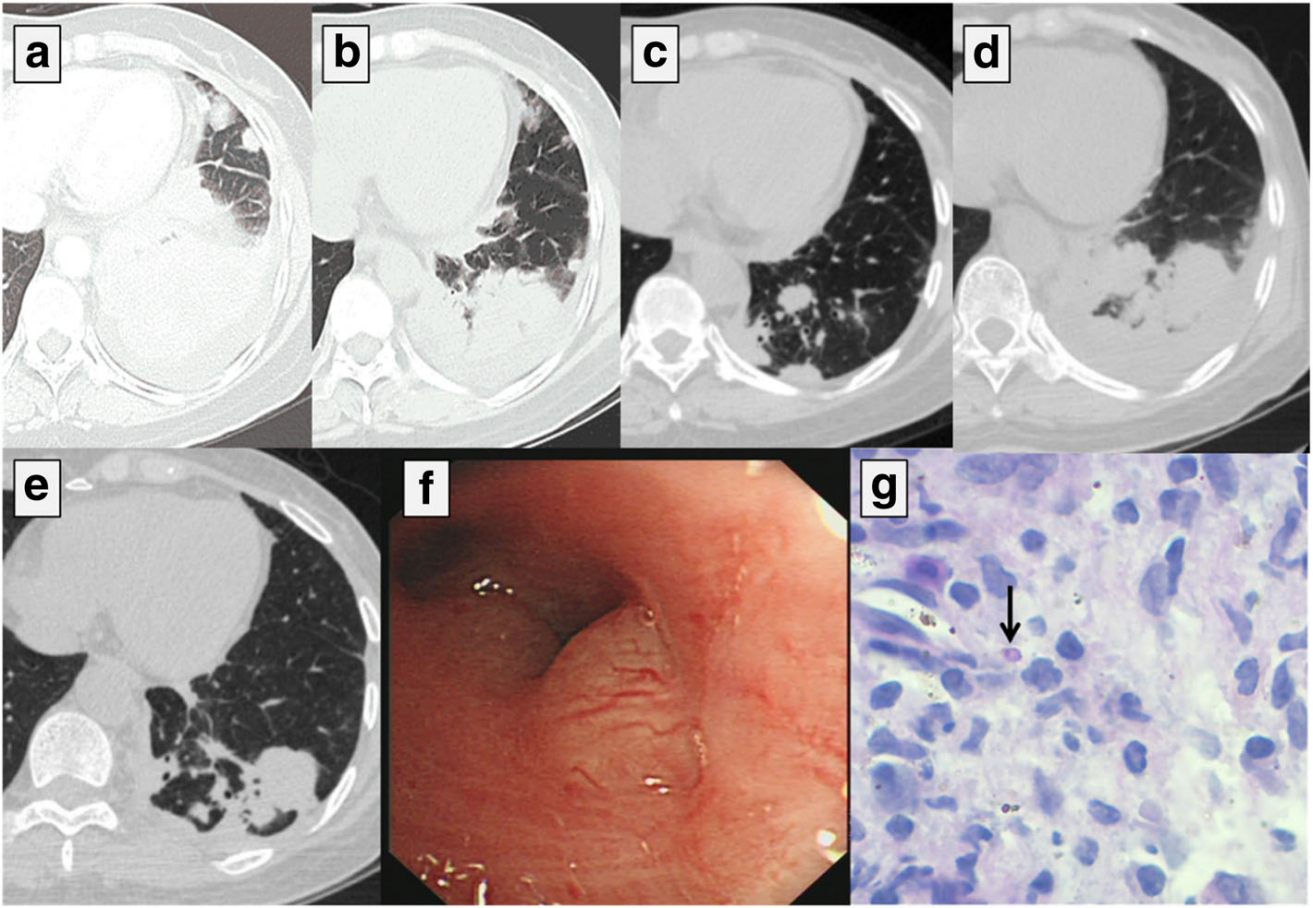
Chest CT (a-e) a) a mass and pleural effusion in the left lower lung on October 28, 2016. b) The mass in the left lower lobe was obviously smaller than that a) on November 2, 2016. c) The size of the nodule in the left lung tended to be stable with little change on April 26, 2017 (seven and a half months after taking apatinib). d) The nodule in the left lung progressed nine and a half months after taking apatinib. e) The lesions significantly improved on September 4, 2018. f) Bronchial stenosis with nodular projections. g) Yeast-like cell could be identified in the endobronchial nodule (PAS original magnification × 1000)
